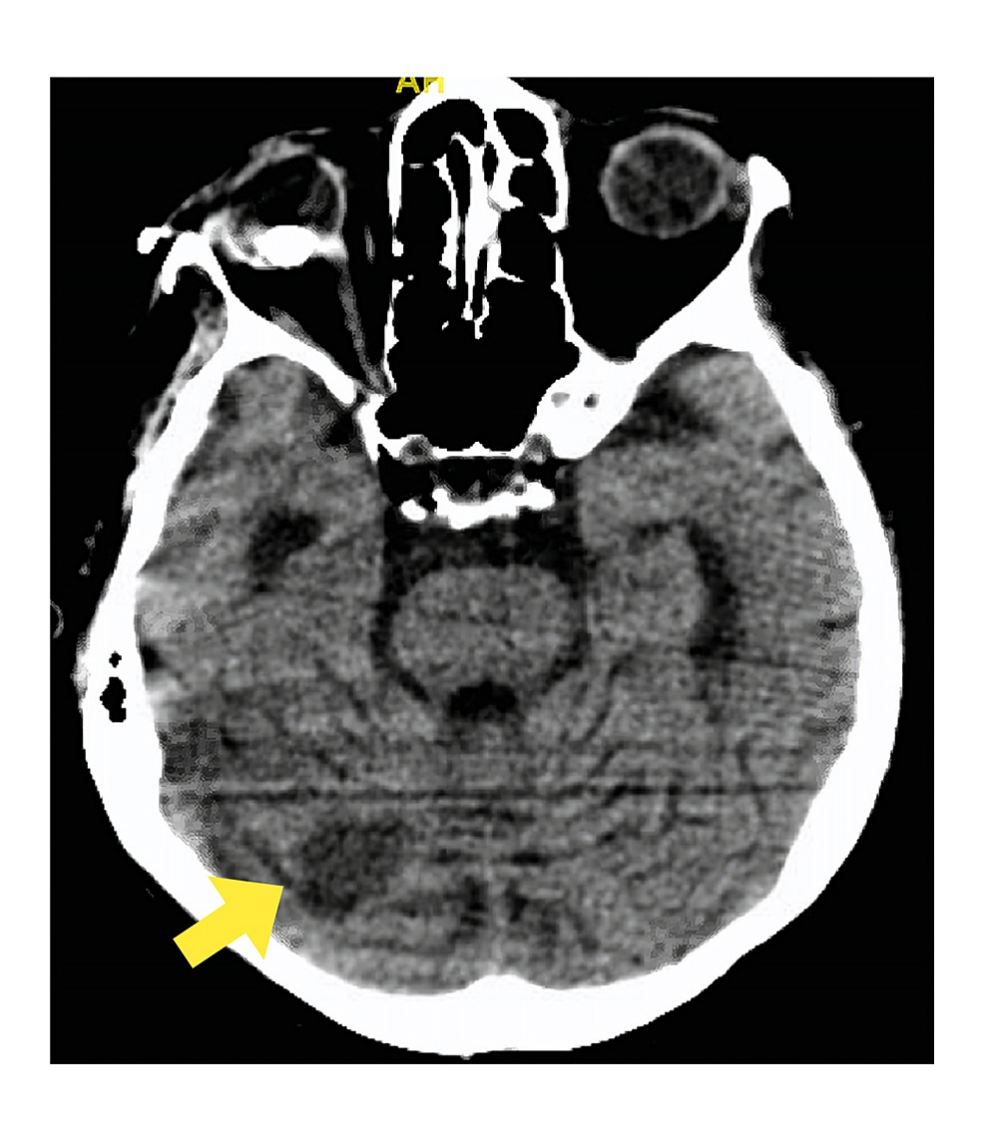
Computed tomography of the brain showing an infarction in the right cerebellum
